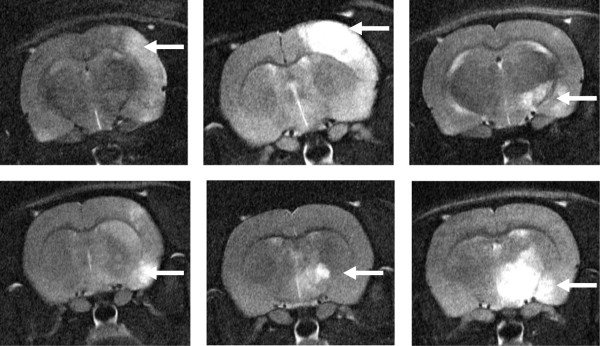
Cerebral MRI. Representative examples of T2-weighted MRI 24 h after embolization of small (1st row) or large (2nd row) bubbles. Note the arrows indicating cortical and subcortical ischemic lesions displayed in white.
